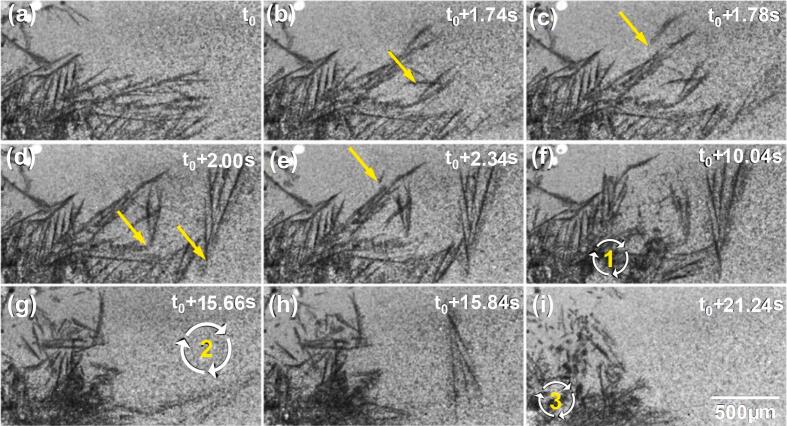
(a-e) Streaming flow induced dendrite fragmentation. (f-i) Swirling flow induced fragmentation. Experimental conditions: 30 W ultrasound, 1137.5 K/m thermal gradient, 250 fps (see Video 4).
